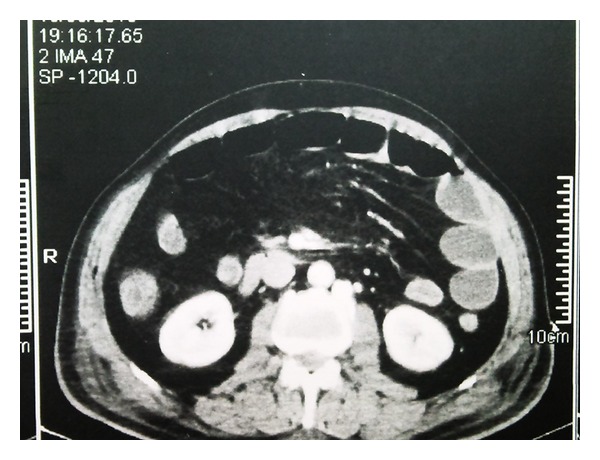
Thickened bowel walls and dilated fluid-filled bowel loops of an AMI patient on an abdominal CT image. AMI: acute mesenteric ischemia; CT: computerized tomography.
